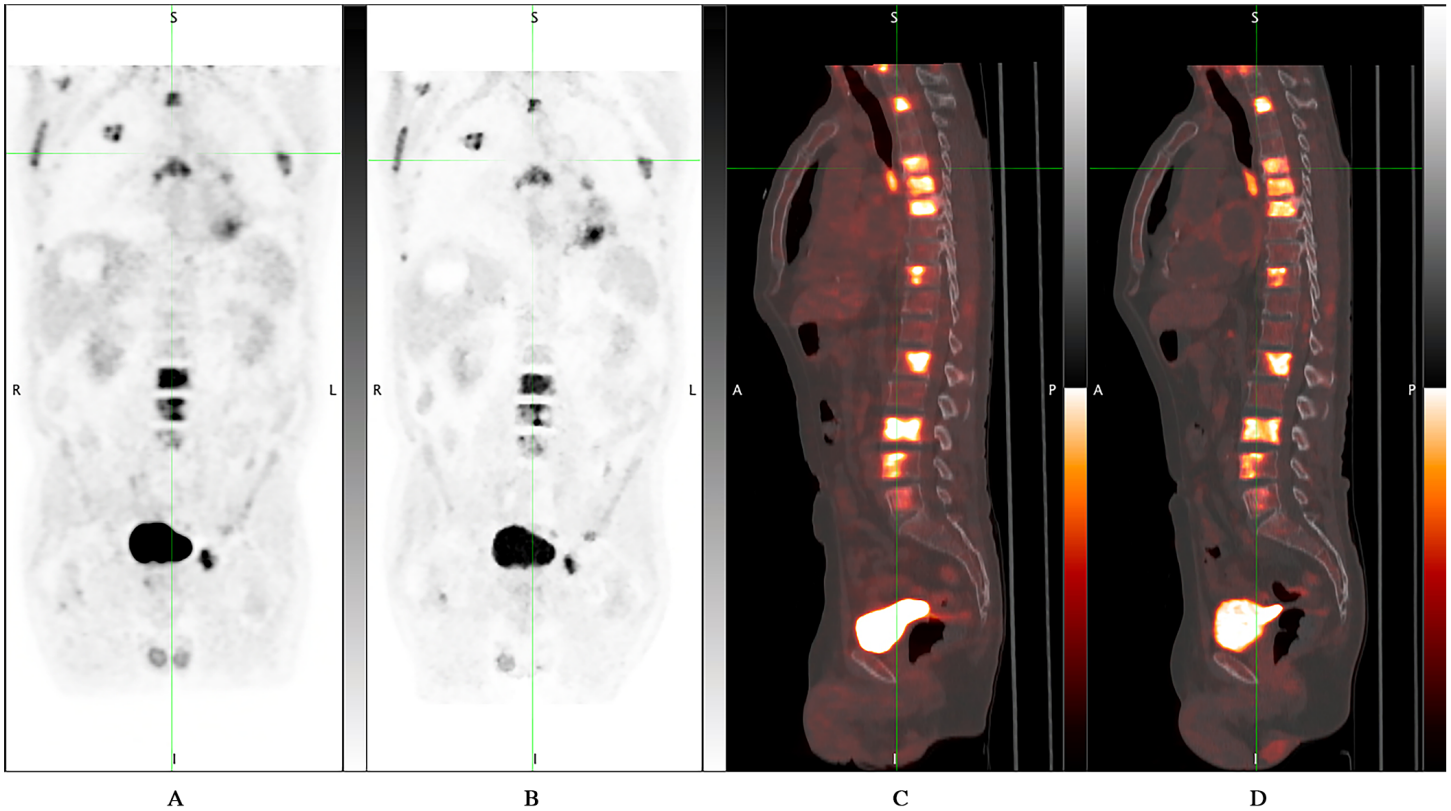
D600 PET/CT vs DMI PET/CT: no additional bone lesions were detected by DMI PET/CT compared to standard PET/CT. 61 years-old man with tonsillar cancer who underwent PET/CT for evaluation of response to therapy. DMI images were acquired 47.4 minutes after standard acquisition and 126 minutes after ^18^F-FDG injection. A) D600 MIP; B) DMI MIP; C) D600 sagittal fused image; D) DMI sagittal fused image.
